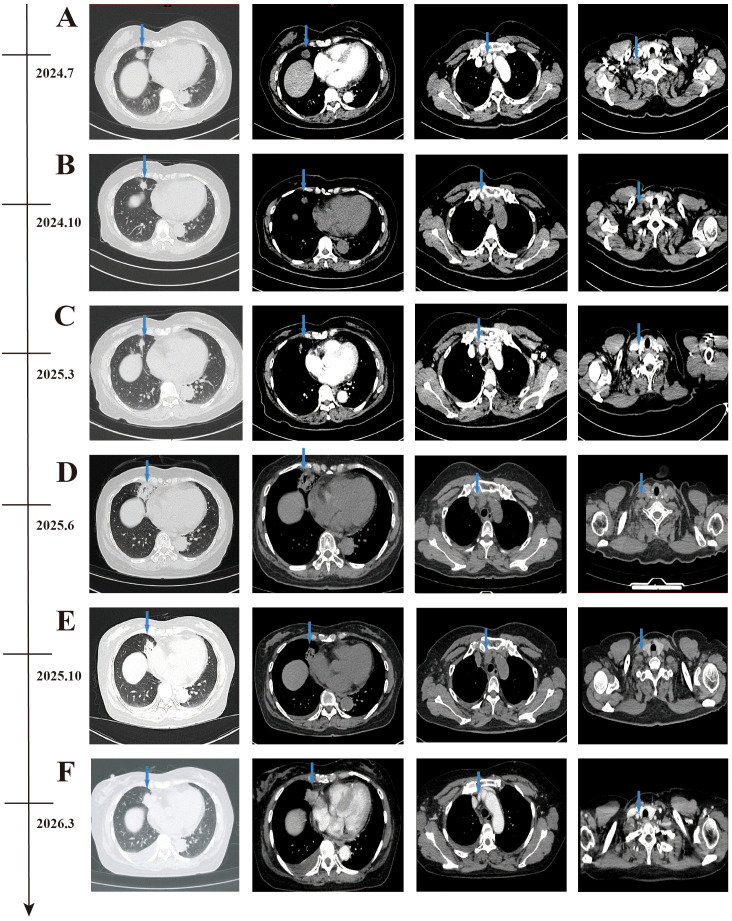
(A) Baseline: Computed tomography (CT) scan of thorax, mediastinal and right supraclavicular lymphadenopathy (24*22mm). (B) CT scan after chemotherapy (18*16mm). (C) CT scan after icotinib plus radiotherapy (16*15mm). (D) CT scan of disease progression (July 2025). (E) CT scan after afatinib therapy. (F) CT scan after pemetrexed + carboplatin + ivonescimab.
